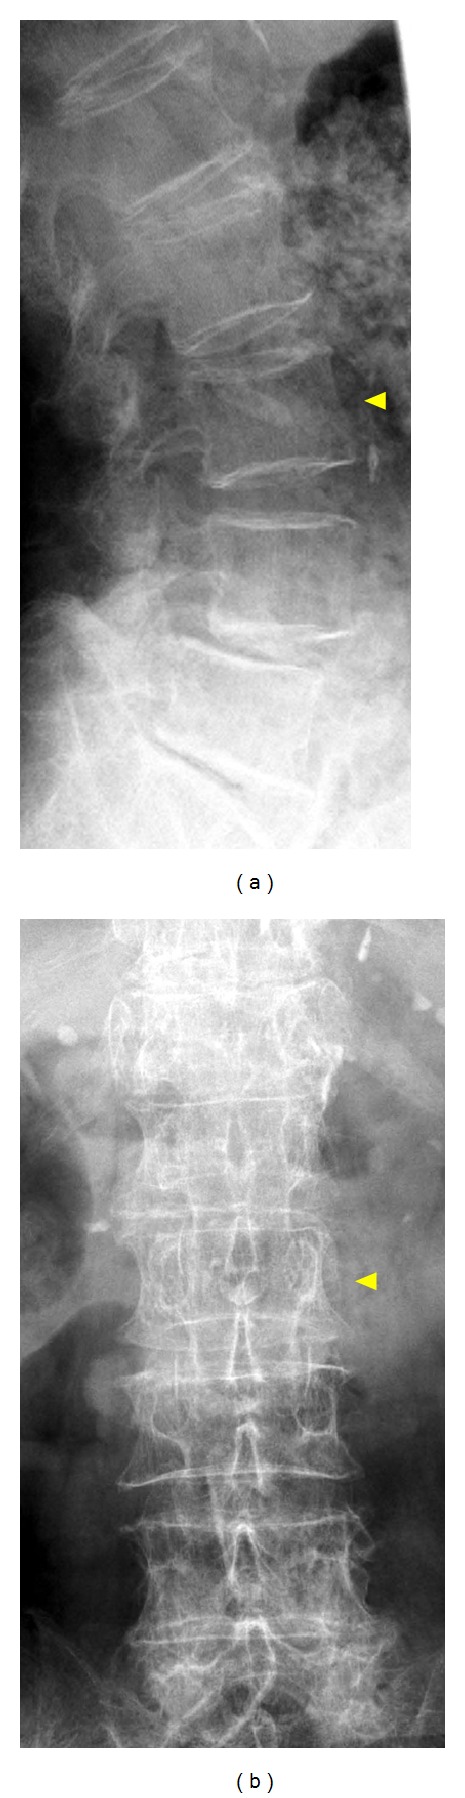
Anteroposterior (a) and lateral (b) radiographs of the lumbar spine of an 85-year-old woman taken immediately after a simple fall, when the fracture in the L3 body (arrowhead) was not obvious.
